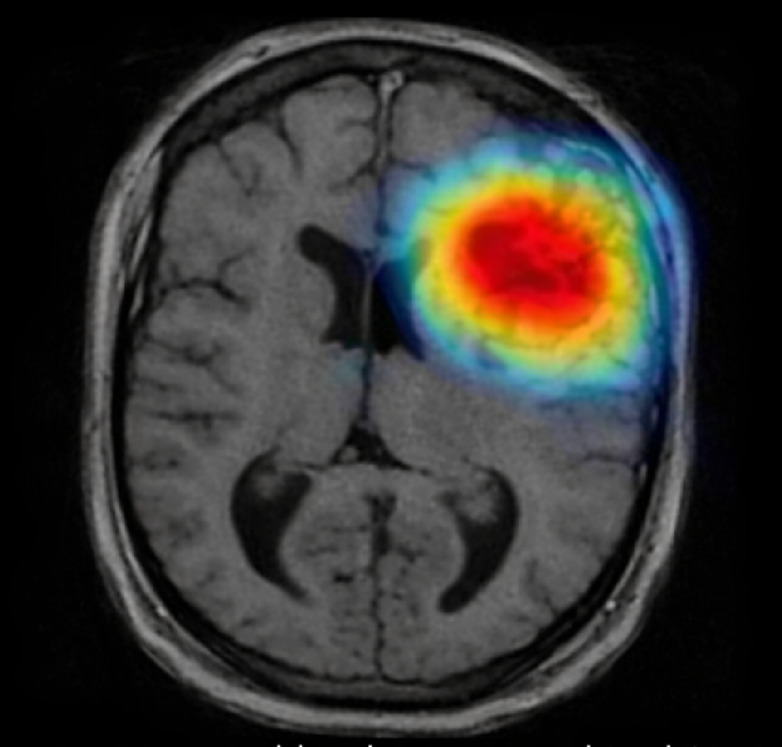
Attention map visualization generated by the proposed MultiAttenNet model highlighting diagnostically relevant tumor regions in MRI images. High-intensity regions (red/yellow) indicate areas of strong model attention corresponding to tumor locations.
